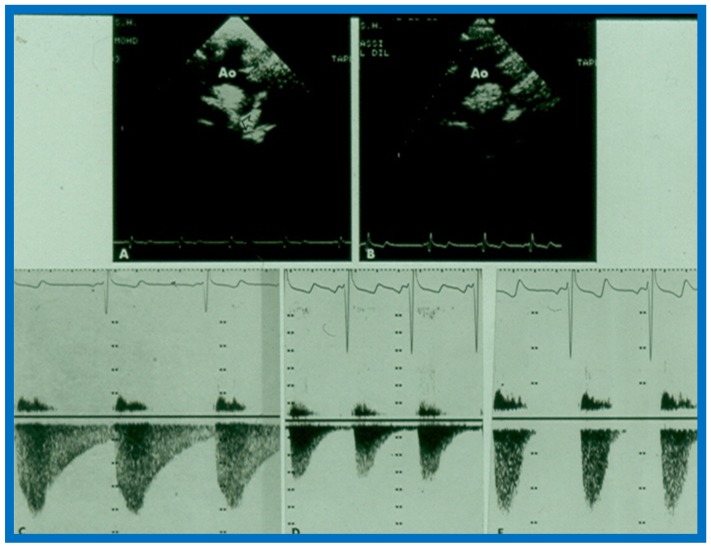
(A,B) Two-dimensional (2D) echo images prior to (A) and following (B) balloon angioplasty of aortic coarctation show improvement in (B). (C–E) Continuous wave Doppler flow velocity recordings from suprasternal notch directing the Doppler signal towards the descending aorta prior to (C) and immediately following (D) balloon angioplasty of aortic coarctation and at six months after angioplasty (E) are shown. Note the reduction of peak Doppler flow velocity from (C) to (D) with further fall in (E). Also note that the diastolic flow is seen throughout the entire diastole (pandiastolic) prior to angioplasty (C), and is seen only is early diastole immediately after angioplasty (D) and at six-month follow-up (E), there was no diastolic flow at all. Reproduced from Rao P.S. [17].
